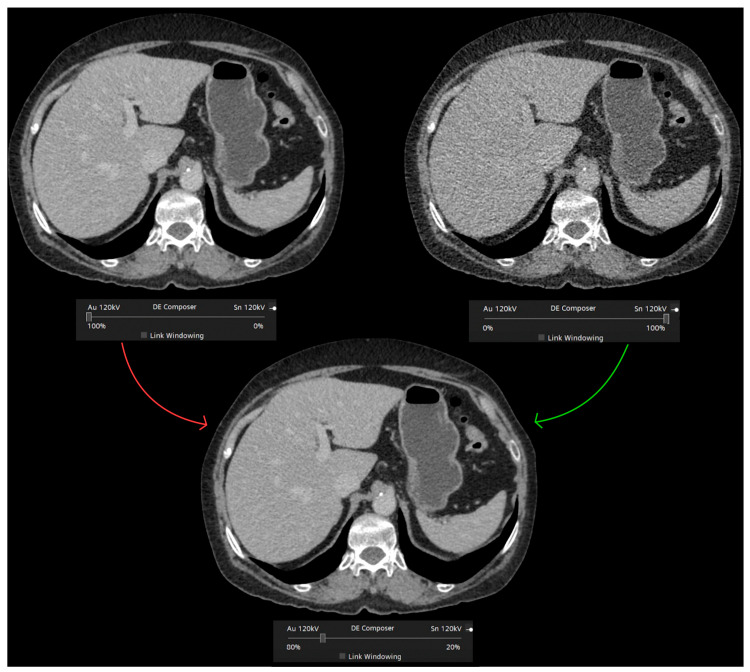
Blended dual-energy image reconstruction. The blended energy values may be chosen by the user, balancing the greater conspicuity of differences in contrast enhancement (at the cost of higher image noise) with low energy values, with the opposite effect of high-energy images. In this example from a TwinBeam DECT scanner (Siemens Healthineers), low- and high-energy beams are derived from a split-filter of gold (Au) and tin (Sn) at the X-ray tube.
